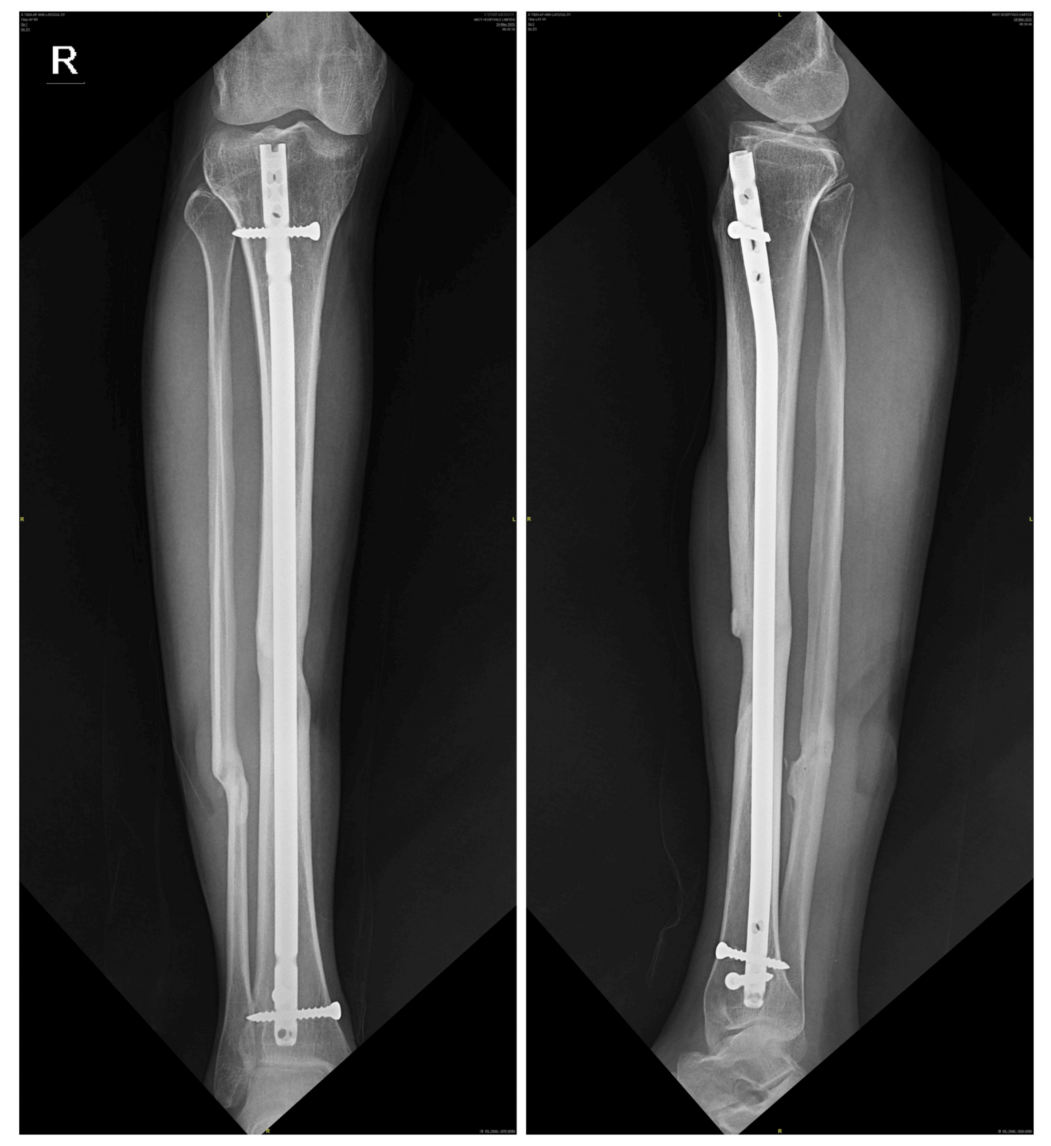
X-ray of the right tibia showing healed shaft of the tibia fracture, five years following cannulated tibial nail (11 mm in size) with one proximal locking screw in dynamic mode and two distal locking screws
